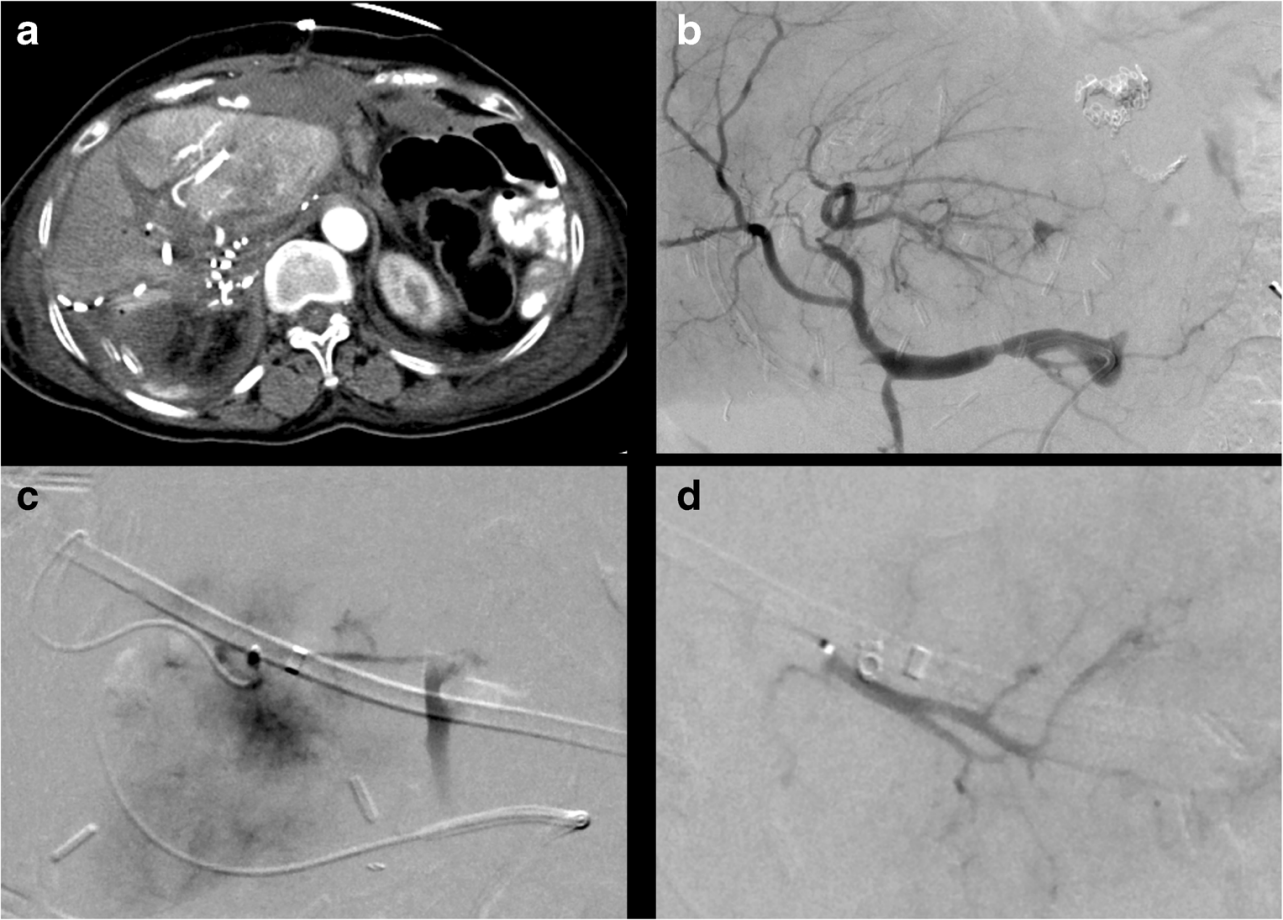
Case of a 66-year-old patient who underwent right hemihepatectomy to treat an intrahepatic cholangiocarcinoma. On day 12 after surgery, the patient developed an insufficiency of the biliodigestive anastomosis, which was treated by PTBD. Two days after the procedure, the patient presented clinical signs of hypovolemia. a CT acquired during the arterial contrast phase exhibited an arterial bleeding with peri-hepatic hematoma along the site of insertion of the PTBD in segment II. b, c DSA revealing the site of bleeding. d DSA after successful transarterial coil embolization of the bleeding
